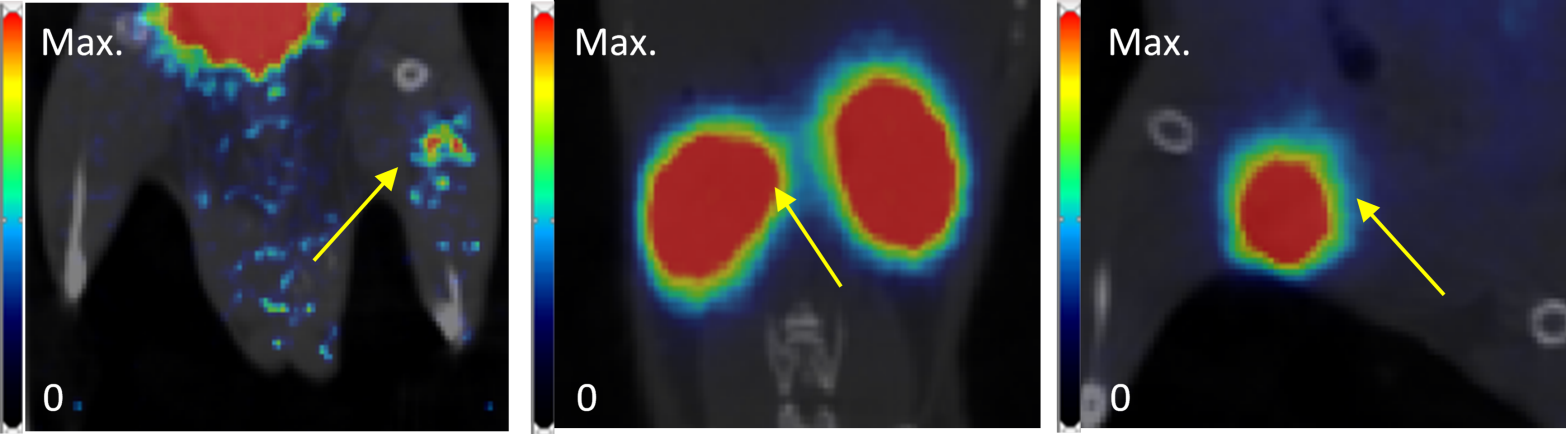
Examples of PET images (coronal slice, frame 30 to 40 min p.i., superimposed on coregistered CT images) of NPICCs in different transplantation sites (left to right: leg, kidney, inguinal fold). Yellow arrow points to the transplantation site.
